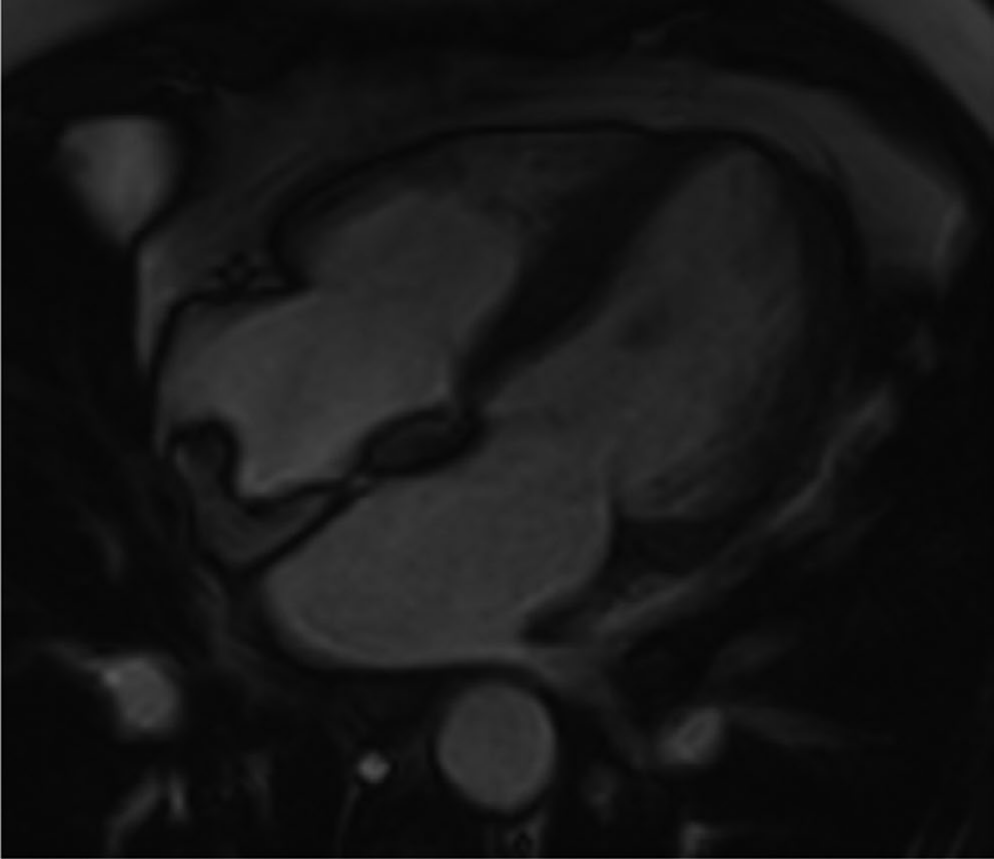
Cardiac magnetic resonance imaging, four‐chamber cine view confirmed the structure attached to the right atrial posterior wall and extends to the interatrial septum
